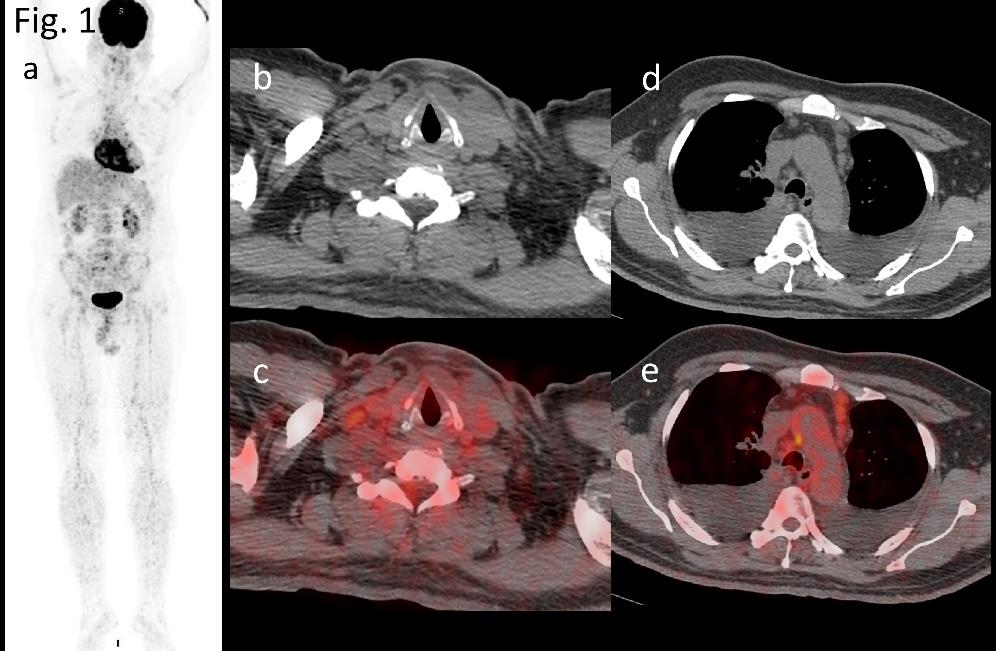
a) 3D MIP of whole body PET CT, b,d) Axial CT and c,e) hypermetabolic right supraclavicular and mediastinal (right paratracheal, pretracheal and left prevascular) nodes
